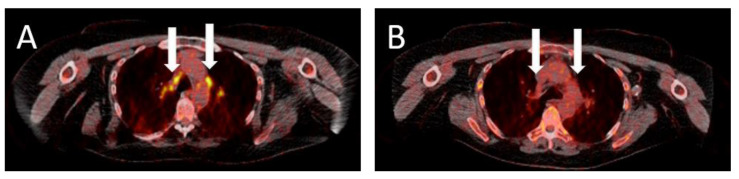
FDG-PET-CT scans of a patient with stage-IV cutaneous melanoma (A,B). The lymph node metastases showed a complete metabolic response following treatment with combined immunotherapy of ipilimumab 3 mg/kg and nivolumab 1 mg/kg every 3 weeks. (A) shows the metastases at the start of immunotherapy. (B) shows the same location after 2 administration cycles. Arrows indicate the location of the (former, B) metastases.
